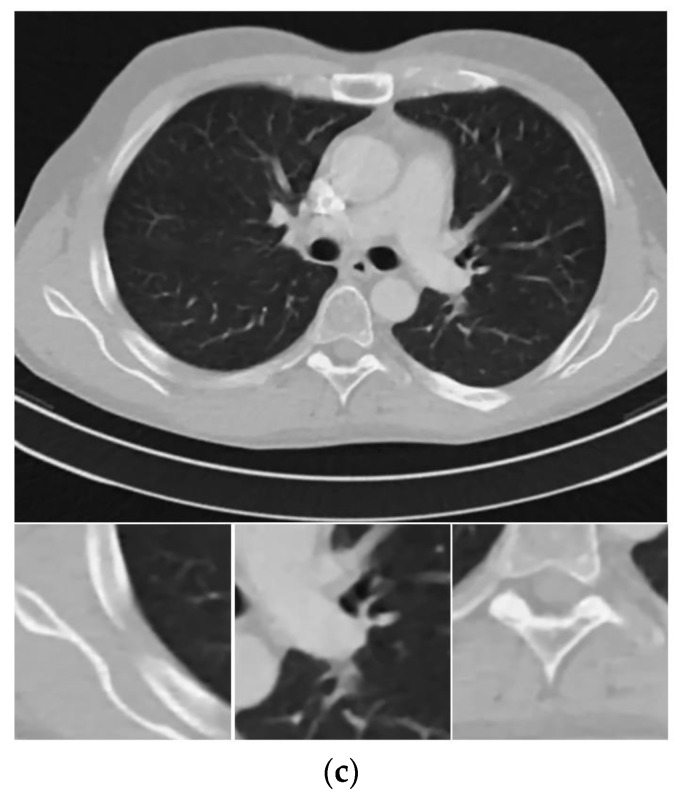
Thorax CT images reconstructed by (a) FBP, (b) SIR-TV, and (c) SIR-STV_1_ methods from noisy projections with 5 × 10^4^ incident photon number. The display window is [−1000, 667] HU.
